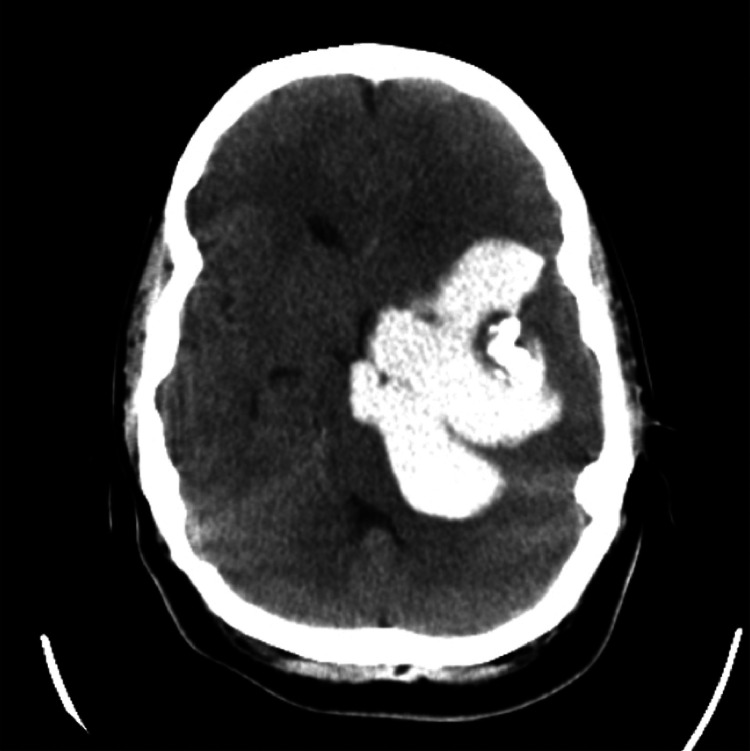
Noncontrast axial brain CT showing an extra-axial, lobulated hyperdense lesion with coarse internal calcifications.
